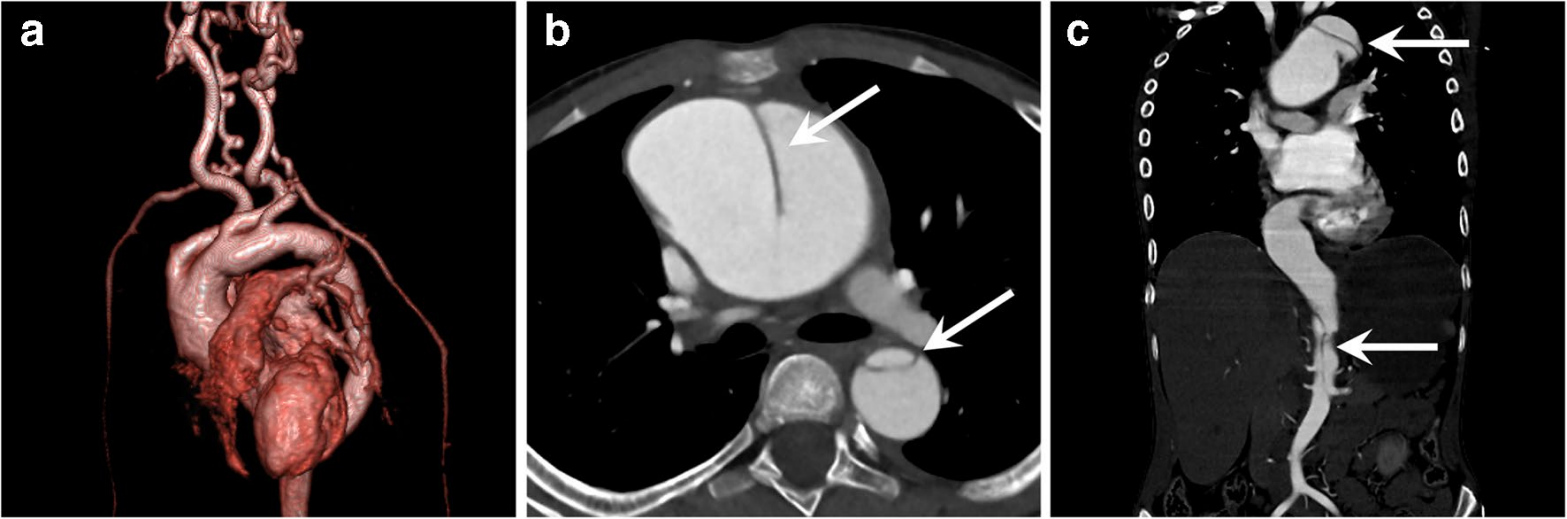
A 4-year-old boy with Loeys-Dietz syndrome complicated by aortic dissection. a Contrast-enhanced computed tomography (CT) parasagittal-oblique volume rendering projection shows a dilated and tortuous aorta, along with severe tortuosity of the head and neck vessels, especially of the vertebral arteries. b, c Axial (b) and coronal (c) CT images 4 years later show an aortic dissection flap (*arrows*) extending from the ascending aorta to infrarenal abdominal aorta, consistent with a type-A dissection
